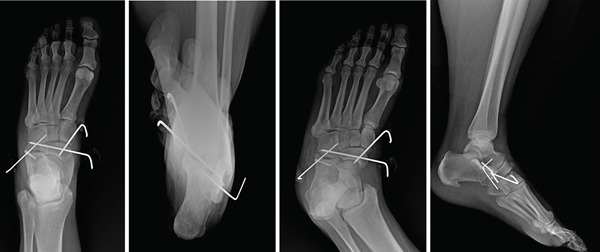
Anteroposterior, oblique, Harris view and lateral radiograph at 2 weeks post‐op.
